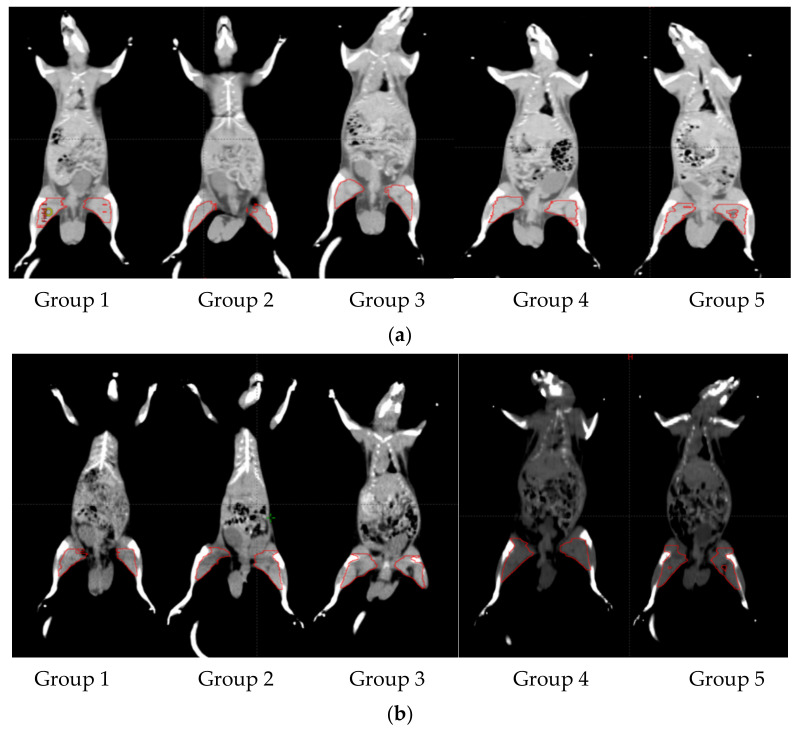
CT muscle scan showing first (a) and last intervals (b).
